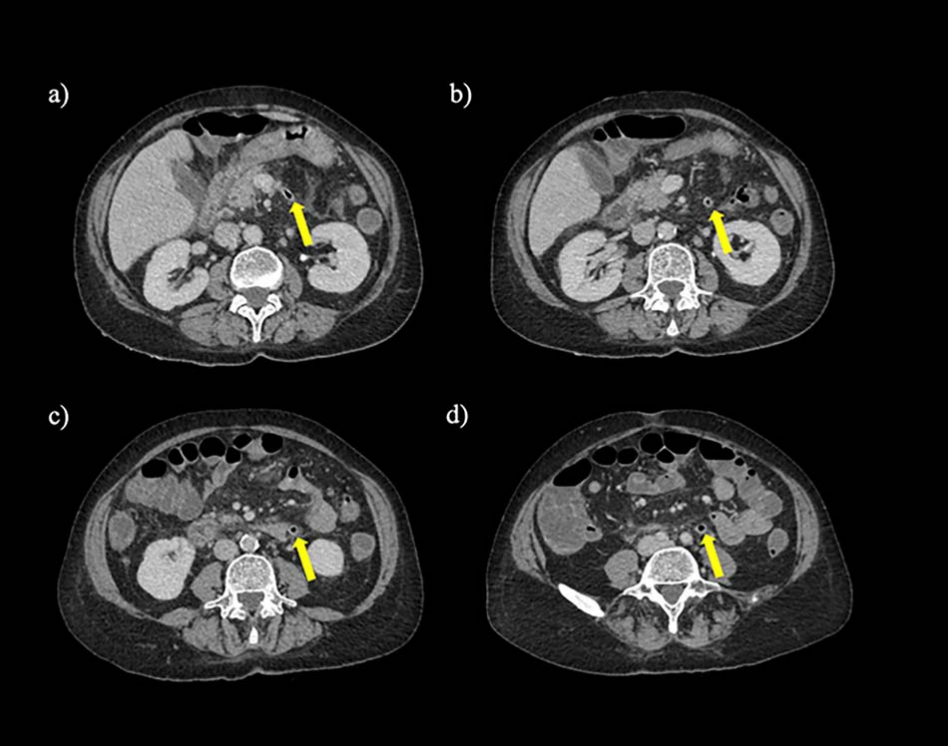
Axial section of a non-contrast CT scan with showing air tracking within the inferior mesenteric vein at various levels (yellow arrow).
